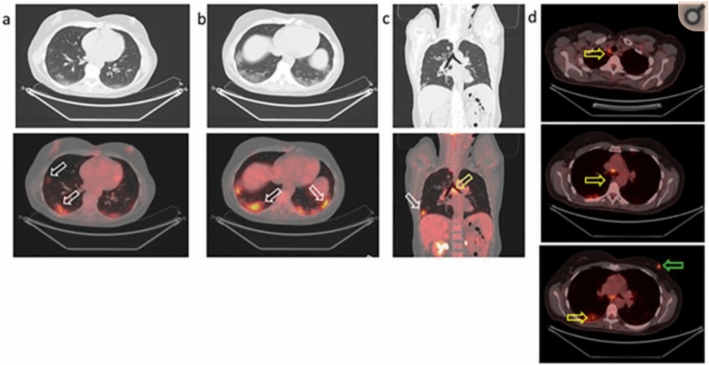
Positron Emission Tomography/Computed Tomography with [18]F-labeled fluorodeoxyglucose (FDG) to restage breast cancer in a 57-year-old woman. Increased Uptake of FDG (white arrows) in subpleural ground-glass opacities of both lungs in the axial (a,b) and coronal (c) section. Tracer uptake in the carinal (c) and mediastinal (d) lymph nodes due to infection (yellow arrows). Focal tracer uptake in the left breast corresponds to the suspected nodule (d; green arrow) (Reproduced from M. Scarlattei et al. [60] under creative commons use)
